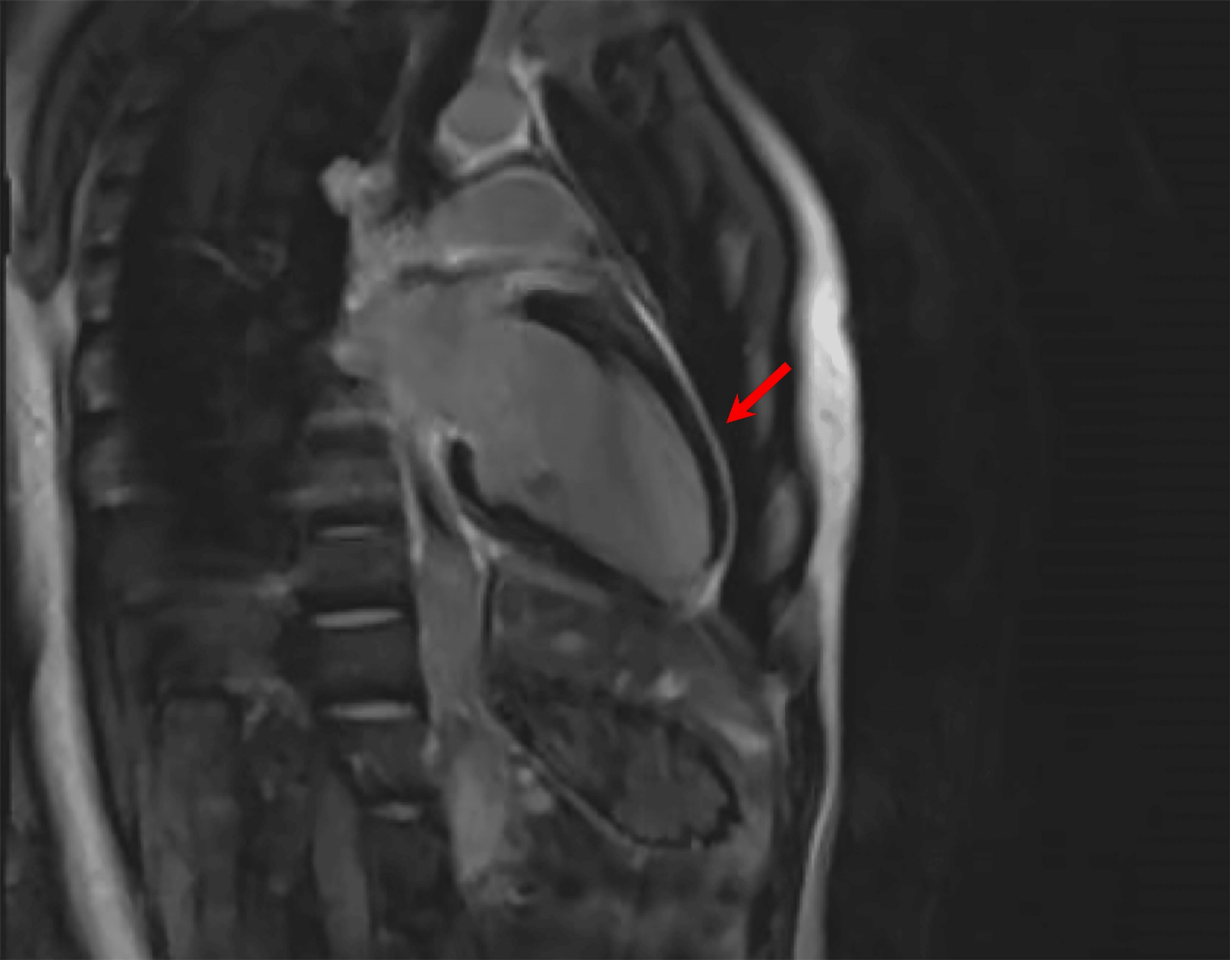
Sagittal cardiac MRI view demonstrating diffuse pericardial enhancement (red arrow) consistent with a diagnosis of pericarditis.
